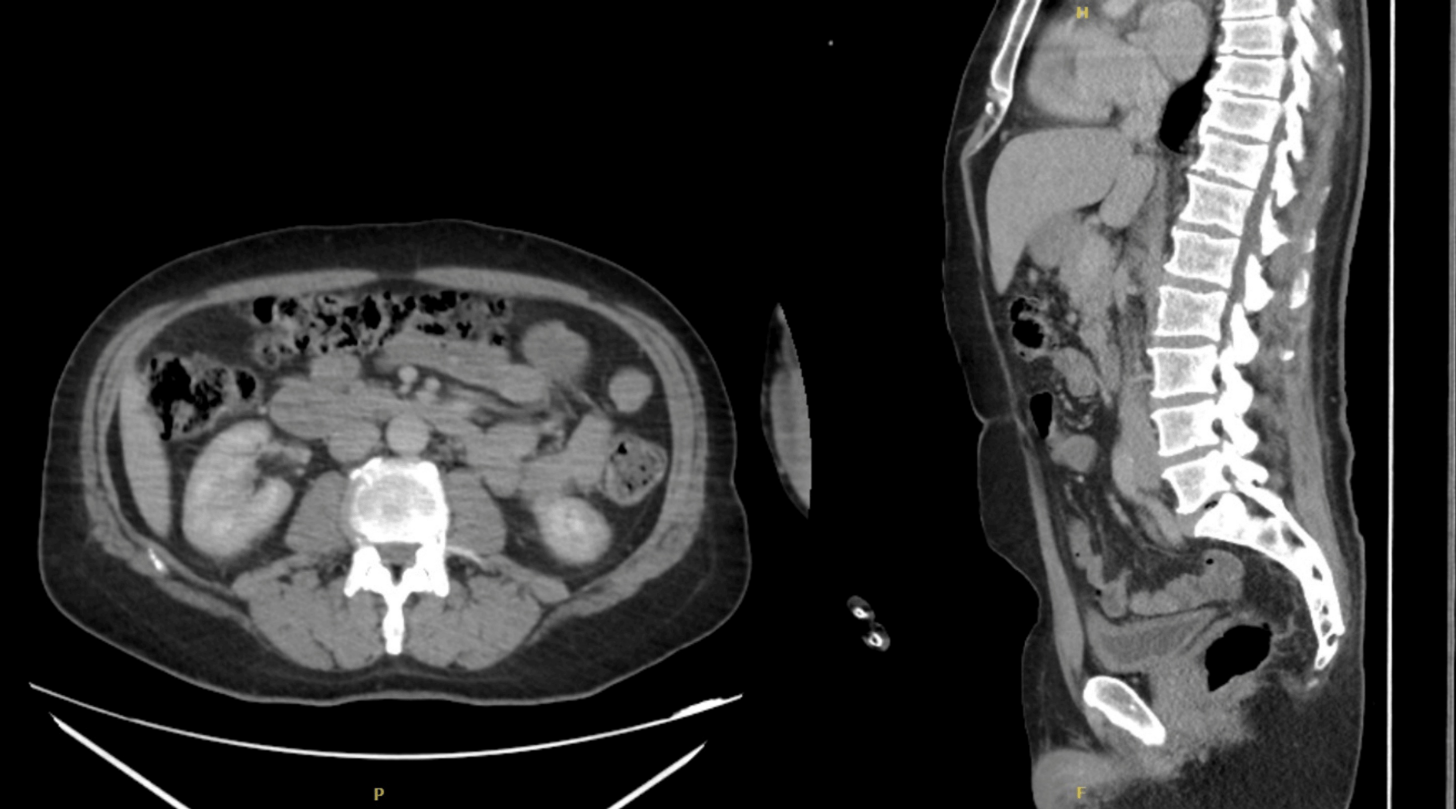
CT of the abdomen in axial (left) and sagittal (right) views showing normal findings. The kidneys, liver, spleen, and pancreas appear unremarkable. Bowel loops are normal, with no signs of obstruction. The spine is well aligned, and no masses or abnormal fluid collections are seen. No signs of malignancy are present. CT: computed tomography
